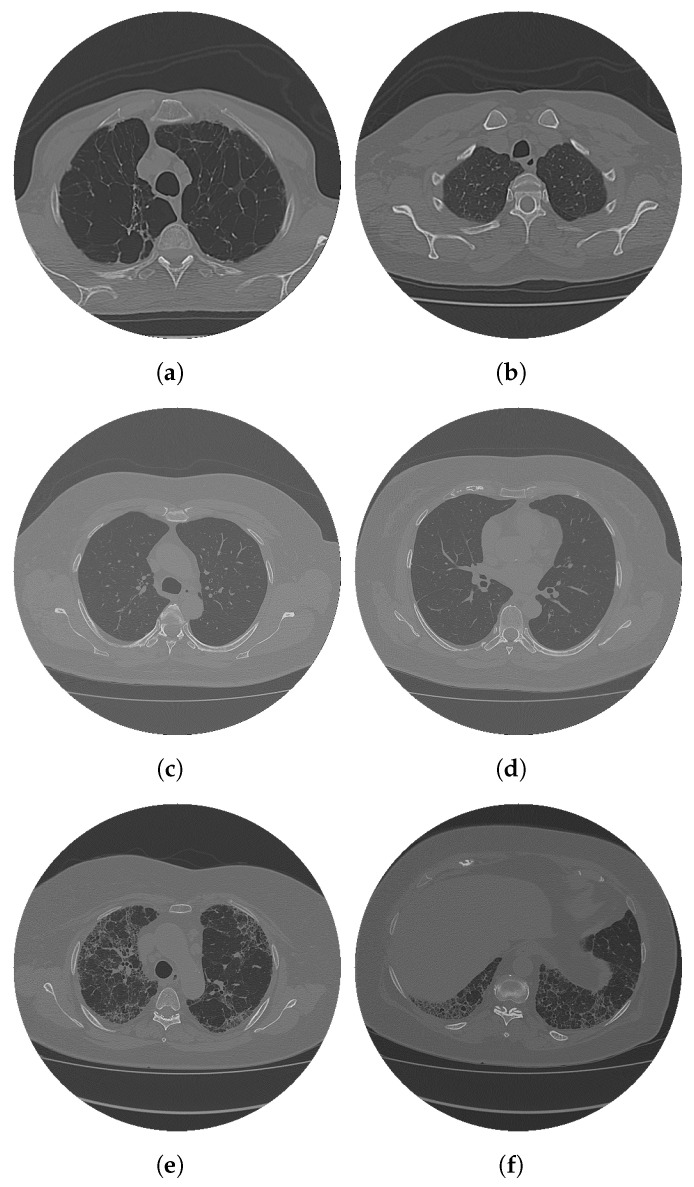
Samples from the chest CT image dataset for lung segmentation. (a) Sample #1 and (b) Sample #4 represent lungs with COPD. (c) Sample #16 and (d) Sample #21 represent healthy lungs. (e) Sample #30 and (f) Sample #36 show lungs with fibrosis.
